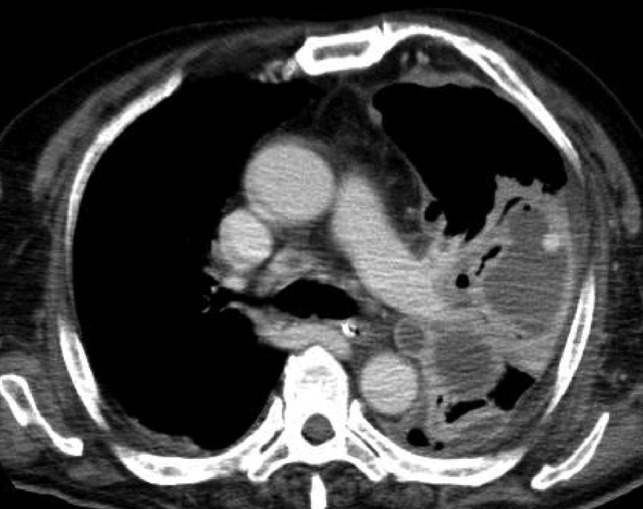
Contrast‐enhanced computed tomography shows a high‐density area within the lung abscess
